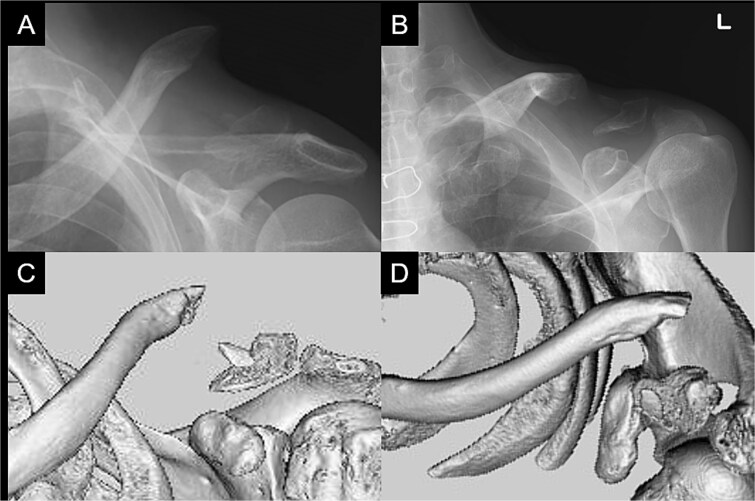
Radiographs (A and B) and CT scans (C and D) captured after 5 months of conservative treatment show that the displacement has progressed.
